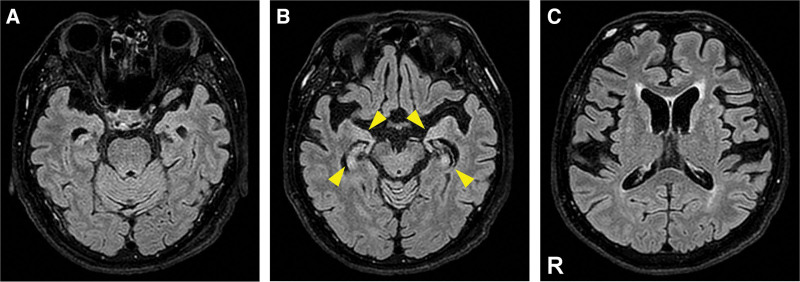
Axial section of T2-FLAIR brain MRI performed on day 75. (A, B) The high-intensity lesions in the bilateral medial temporal lobes on T2-FLAIR brain MRI performed on day 75 partly improved, with weak abnormal signals remaining (indicated by yellow arrows heads). (C) No newly abnormal signals were detected in other limbic area, such as the insula. MRI = magnetic resonance imaging, T2-FLAIR = T2-weighted fluid-attenuated inversion recovery.
